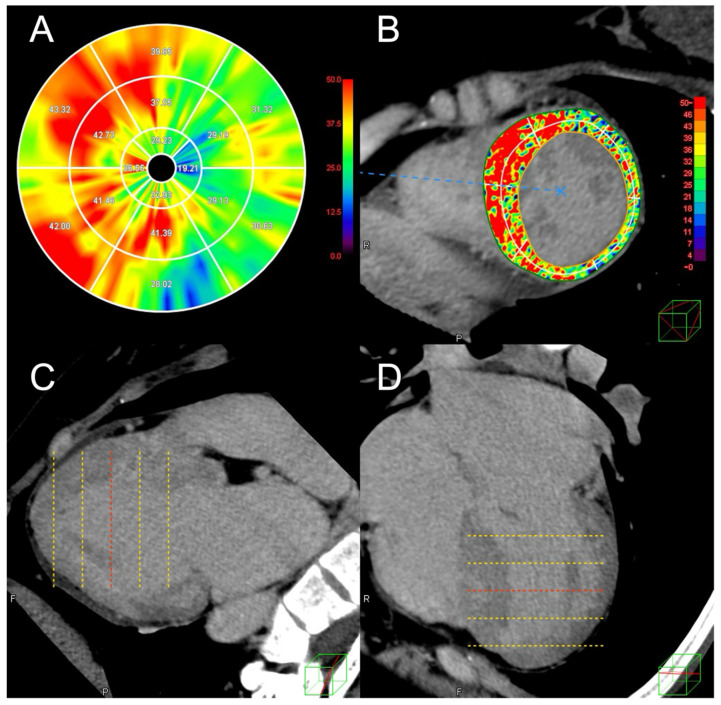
Extracellular volume analysis of left ventricular myocardium on computed tomography images in a case with hypertrophic cardiomyopathy. (A) A polar map displaying the segmental extracellular volume fraction (ECV) of left ventricular myocardium for each of the 16 American Heart Association segments. (B) A short-axis view with a color-coded ECV map overlaid. (C) Two-chamber and (D) four-chamber views of the late-phase computed tomography (CT) images used for the analysis. This case is of a 28-year-old female with HCM who was hospitalized for heart failure after the CT scan. The overall ECV of the left ventricular myocardium on CT was 37.6%.
